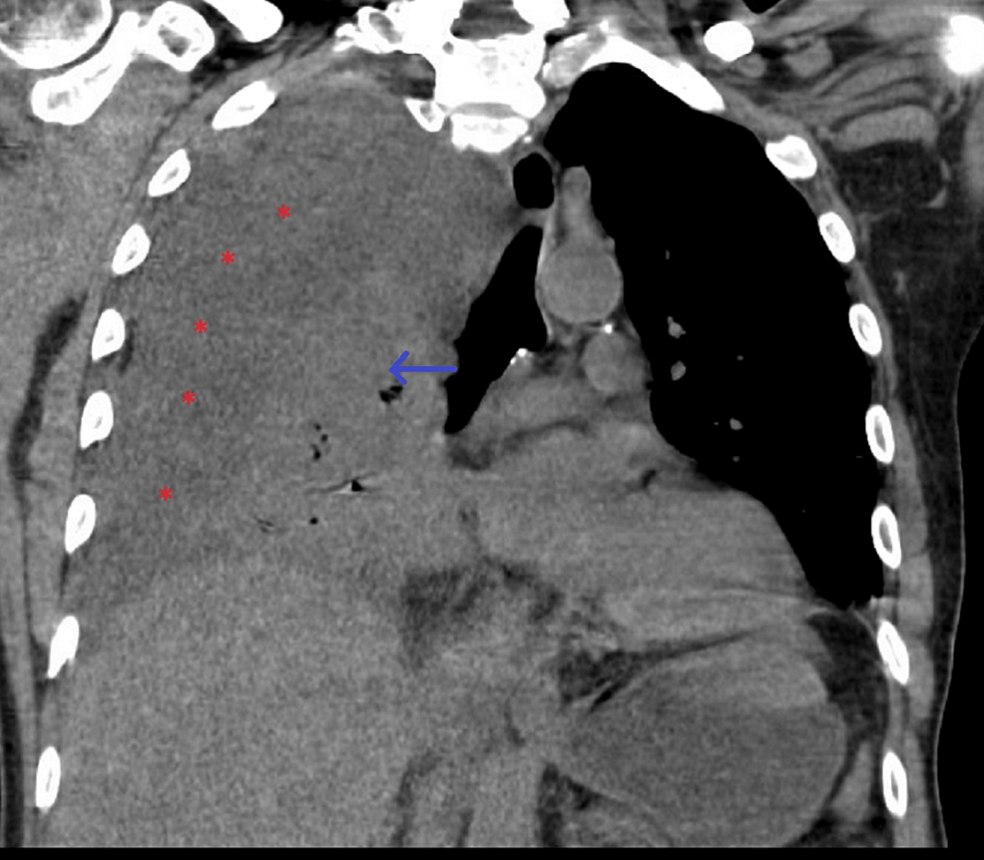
CT of the chest without contrast, coronal cuts, showed large right pleural effusion (red asterisk) with extensive atelectasis and consolidation of the right lung (blue arrow).
